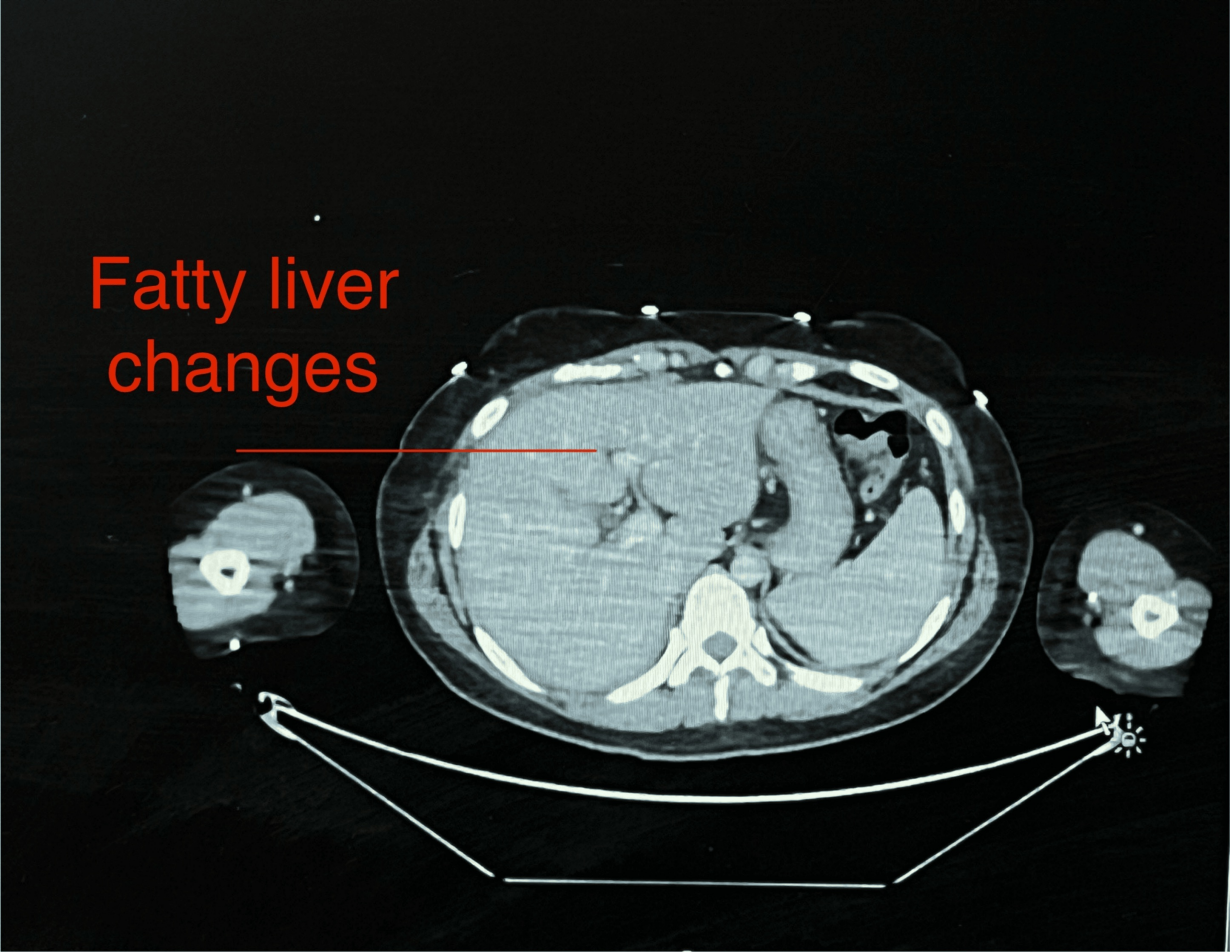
CT Images showing fatty liver .
